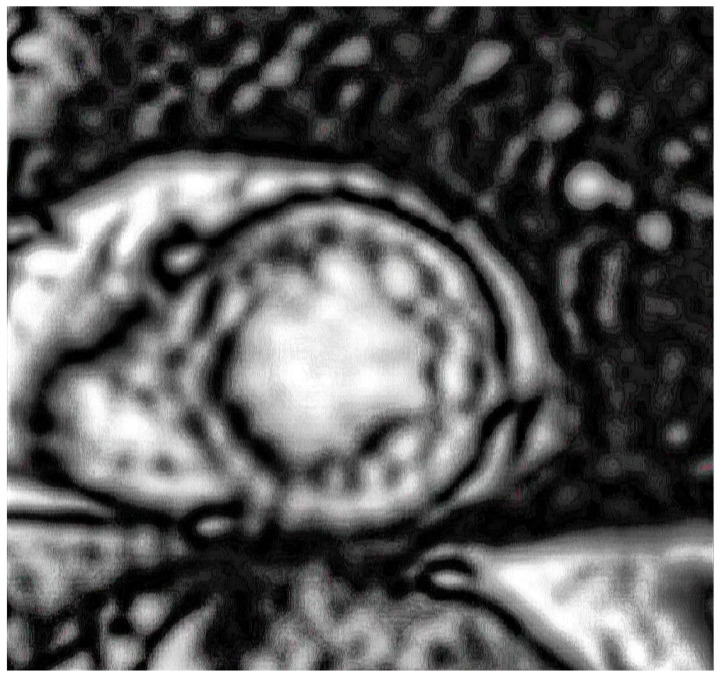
The stress MRI reveals a perfusion defect localized to the apical segments of both the septal and inferior walls.
